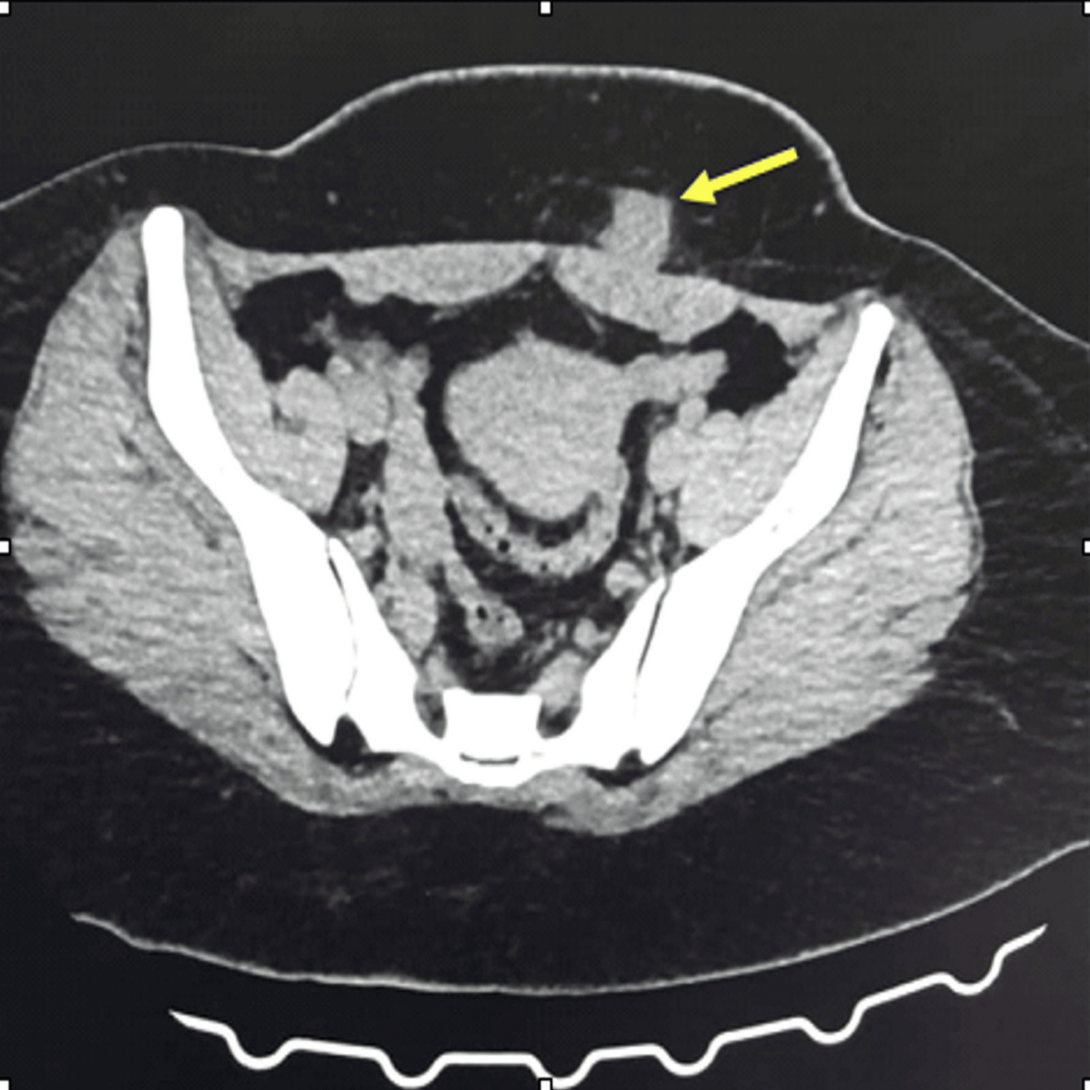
CT scan of the pelvis shows the lump at the lateral third of the scar site anterior to the left rectus muscle.
